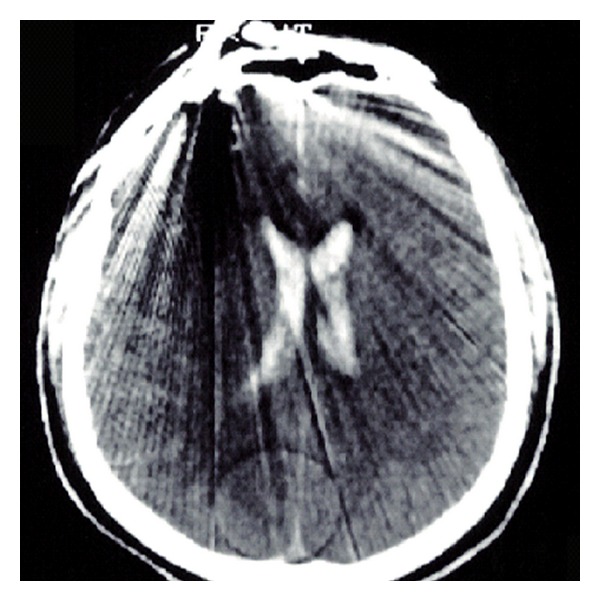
Preoperative axial CT scan of the head shows the entry point of the metallic body, causing radiographical artifacts. The frontal horn damage can be suspected because of the bilateral intraventricular hemorrhage.
